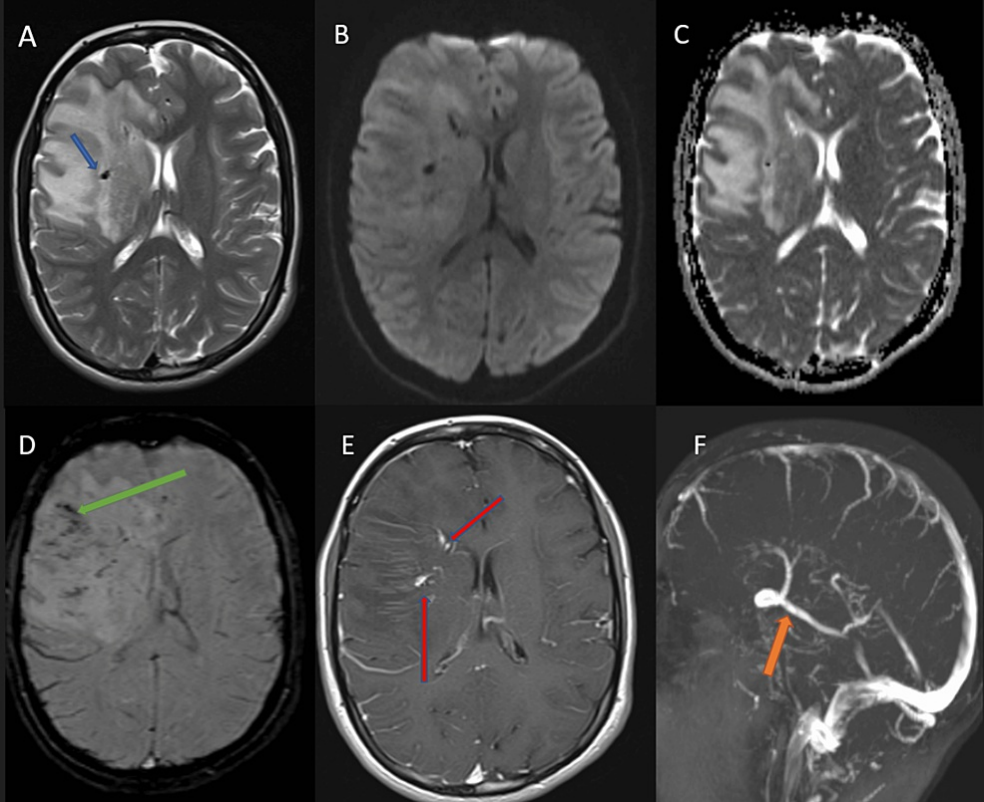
Brain MRI of the patient performed 24 hours after symptom onset The T2 weighted axial image (A) showed extensive vasogenic edema in the right frontal lobe with a mass effect. A vascular flow void (arrow) was noted within the edematous region. Diffusion-weighted imaging (DWI) (B) and apparent diffusion coefficient (ADC) axial (C) images showed no diffusion restriction. The susceptibility-weighted image (D) and post-gadolinium T1 axial image (E) showed abnormal veins forming a radial array of “caput medusae” appearance (red arrow), converging to the beginning of the dilated collector vein. The collector vein was patent in the MR venogram (F), coursing back along the brain surface, and draining into the right transverse sinus.
